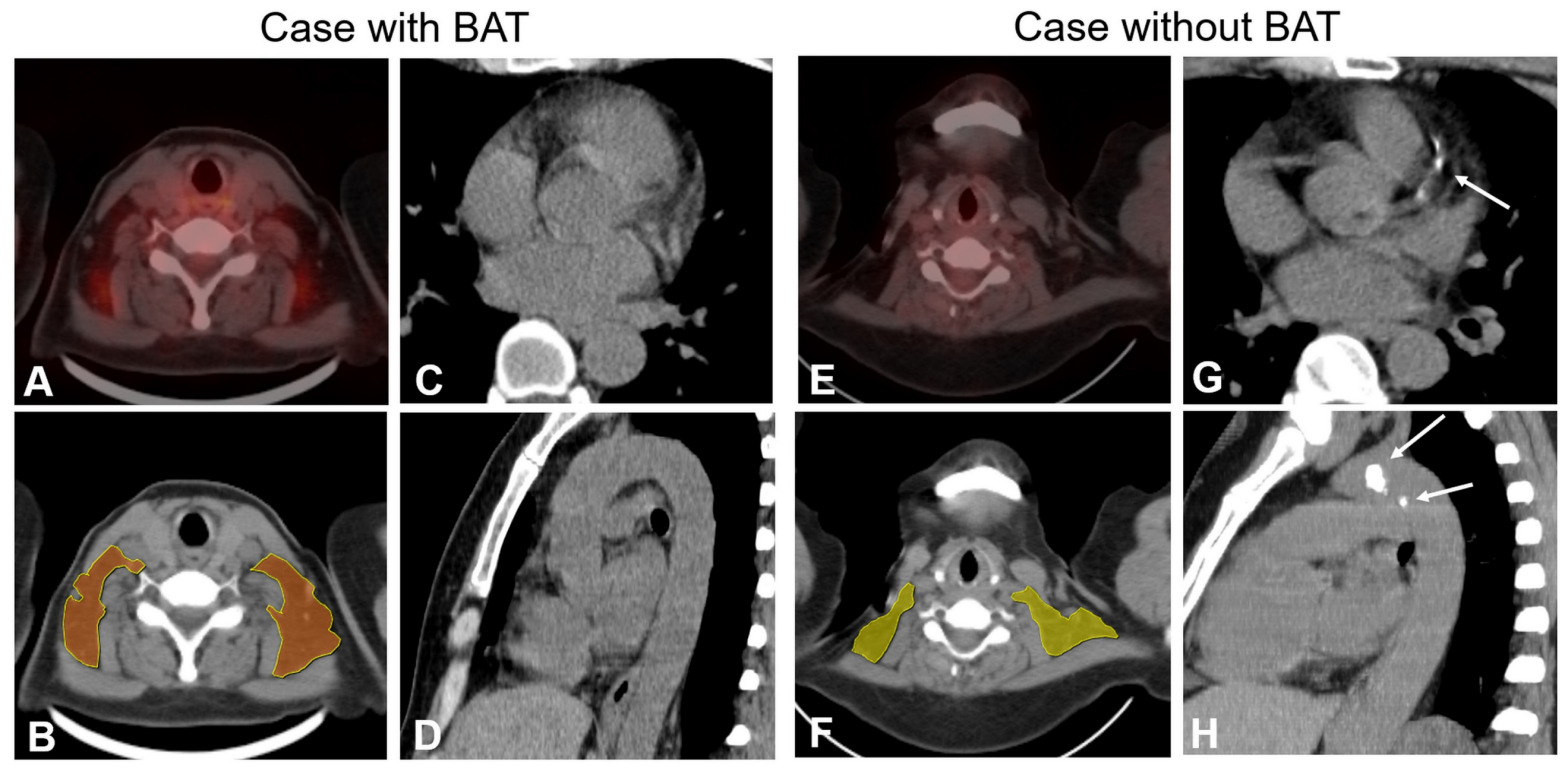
Examples of patients with and without BAT and cardiovascular calcification. PET-CT finding (panel A) and radiomics model (panel B) show BAT in bilateral supraclavicular depots in a 65-year-old female. Axial and sagittal CT images (Panels C and D) show no calcification of coronary arteries and thoracic aorta. PET-CT finding and radiomics model (Panels D and F) show no BAT depots in a 51-year-old female. Axial and sagittal CT images (Panels G and H) show calcified plaques both in the coronary arteries and thoracic aorta (white arrow).
